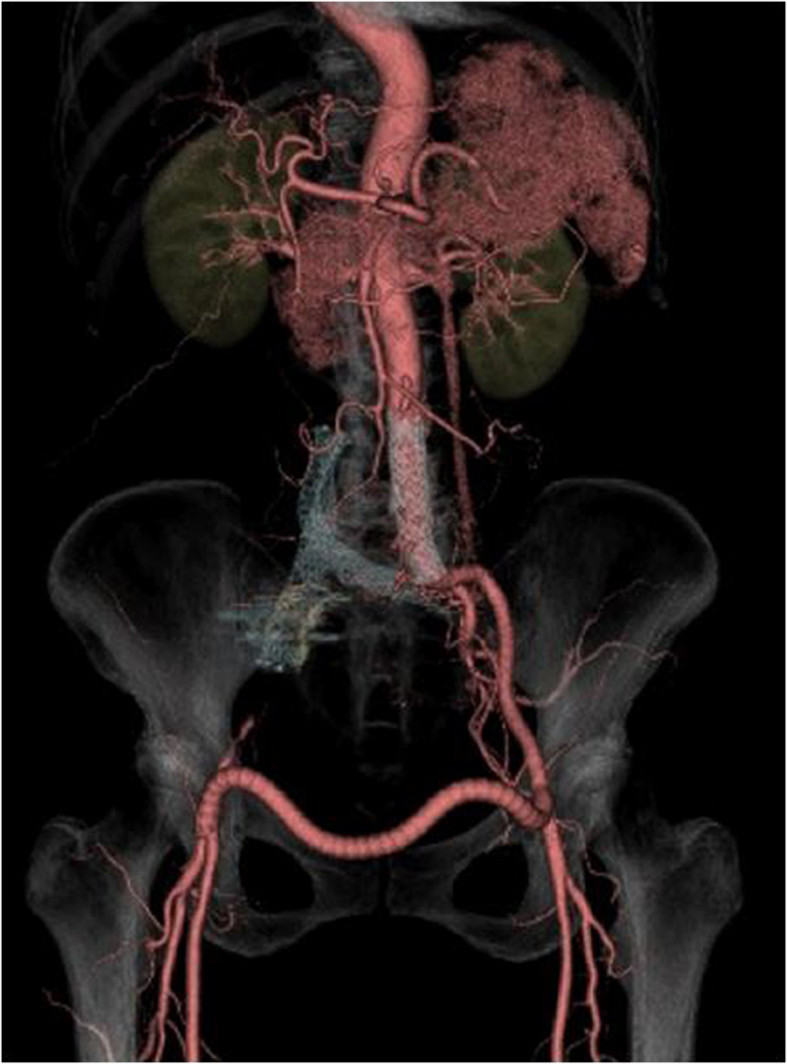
Postoperative 3D CTA, anterior view. There is no endoleak in the stent graft. The fistulous flow has also disappeared. The left external iliac artery to the right common femoral artery (I–F) bypass flow shows good patency.
